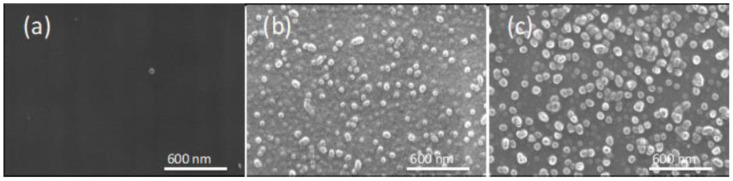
Surface SEM images of dry PIMs with a composition of 60%IL–40%CTA. (a) AlqCl; (b) AlqNO_3_; and (c) AlqSCN.
